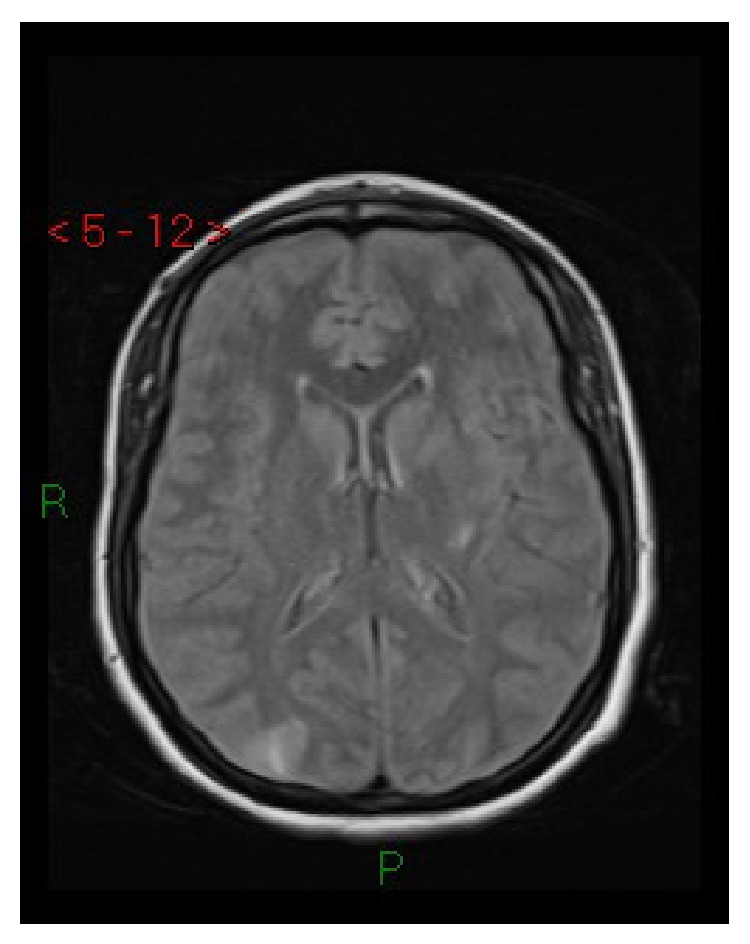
Case 1. Axial FLAIR MRI: cortical-subcortical hyperintense lesions in parietal-occipital regions and in the posterior lateral left putamen.
